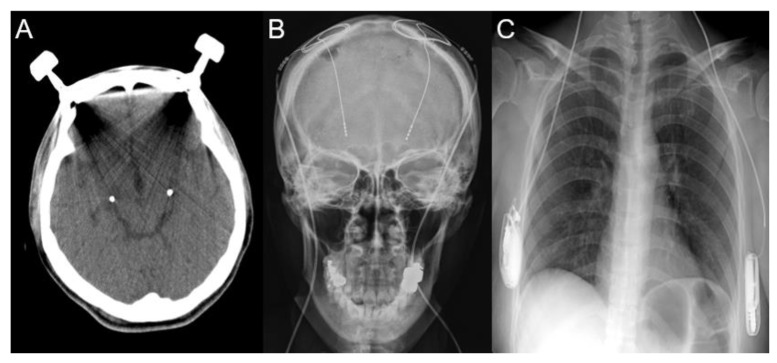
(A) Postoperative computed tomography (CT) scans revealed bilateral deep brain stimulation (DBS) electrodes implantation in the globus pallidum internus (Gpi) without any surgery-related complication. (B,C) 10 days after DBS, implantable pulse generators (IPGs) were implanted subcutaneously in the sub-axillary region.
